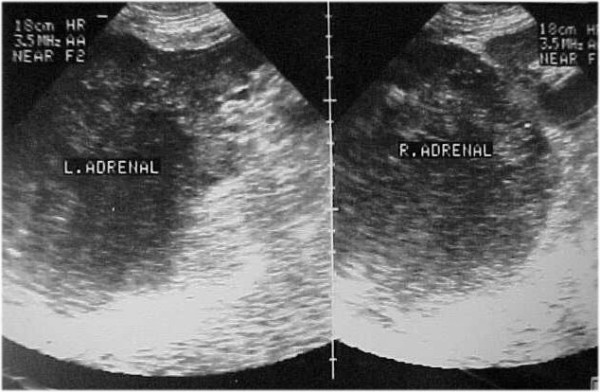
Abdomen ultrasound showing large masses with foci of hemorrhagic necrosis in both adrenal glands.
